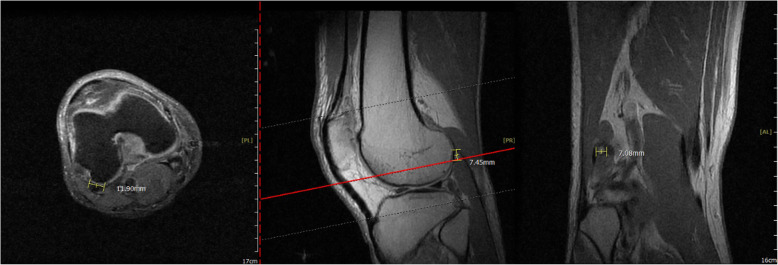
The right knee MRI showed a fabella with subchondral cyst formation in Coronary view
